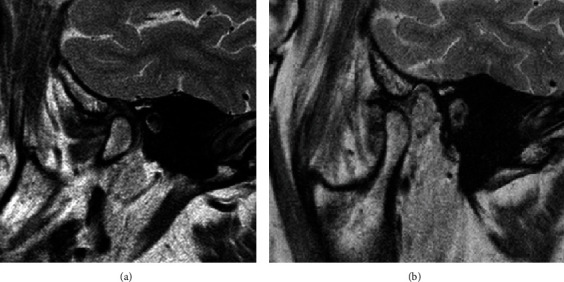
Dynamic MRI of the TMJ at 10-week follow-up with patient's mouth closed (a) and open (b).
